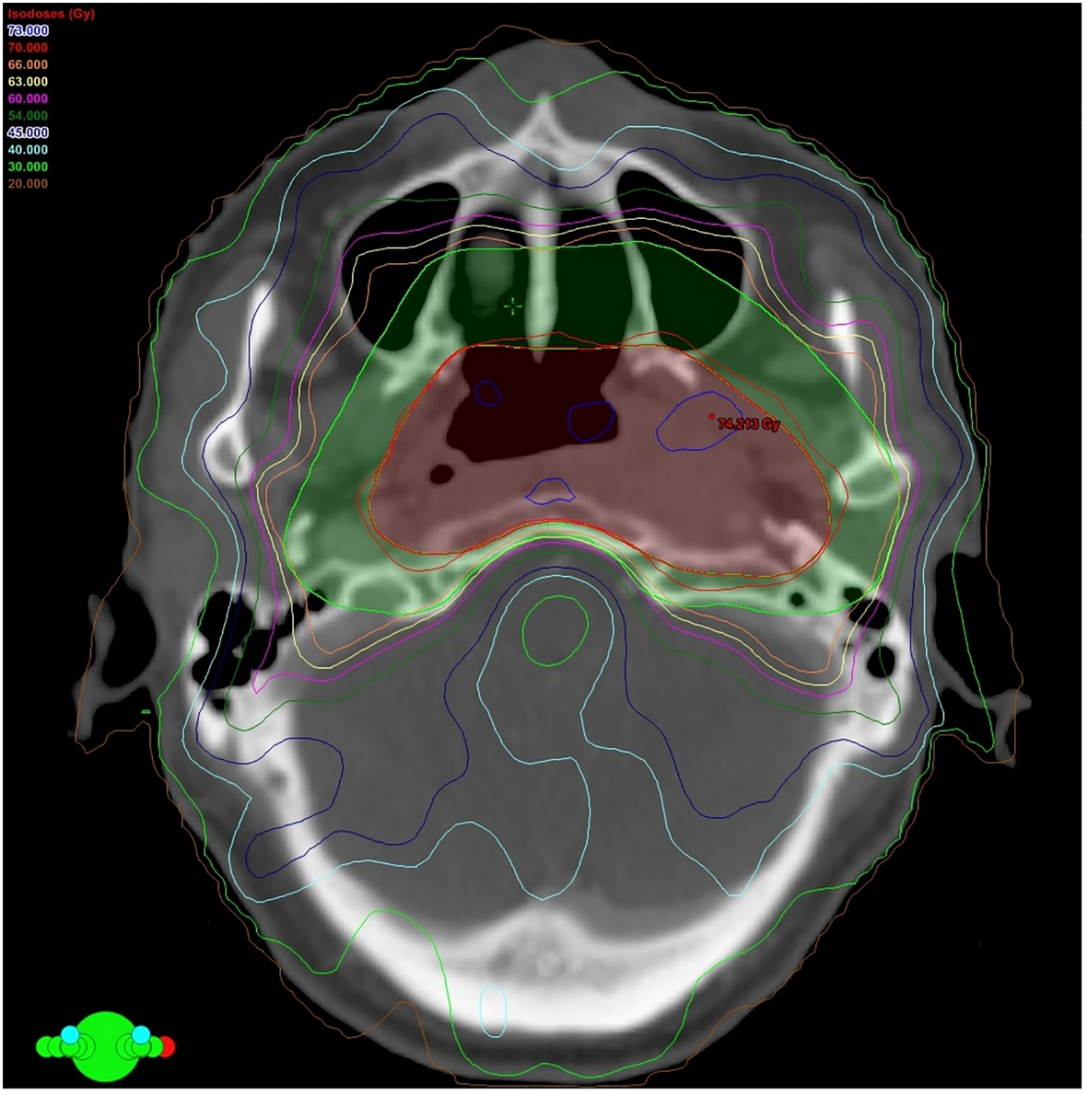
Computed tomography image showing the beam orientation and isodose distribution of a 9-field intensity-modulated radiation therapy (IMRT) plan for a patient with non-metastatic nasopharyngeal carcinoma.
